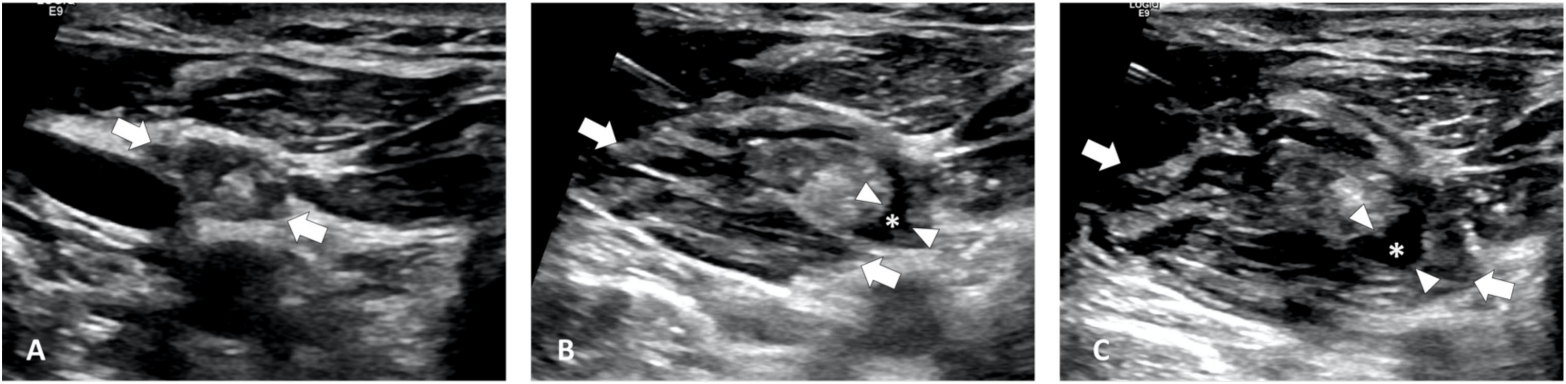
Ultrasound Image of continues hydrodissection. (A) Pre-MWA, B-mode ultrasonography (US) showed hypoechoic LN (white arrows). (B, C) Under continues injection, the NS solution inside PLNS (asterisks, * between white arrowheads) diffuse to outside of LNM capsule to surrounding spaces (white arrows), further pushing important structures away.
